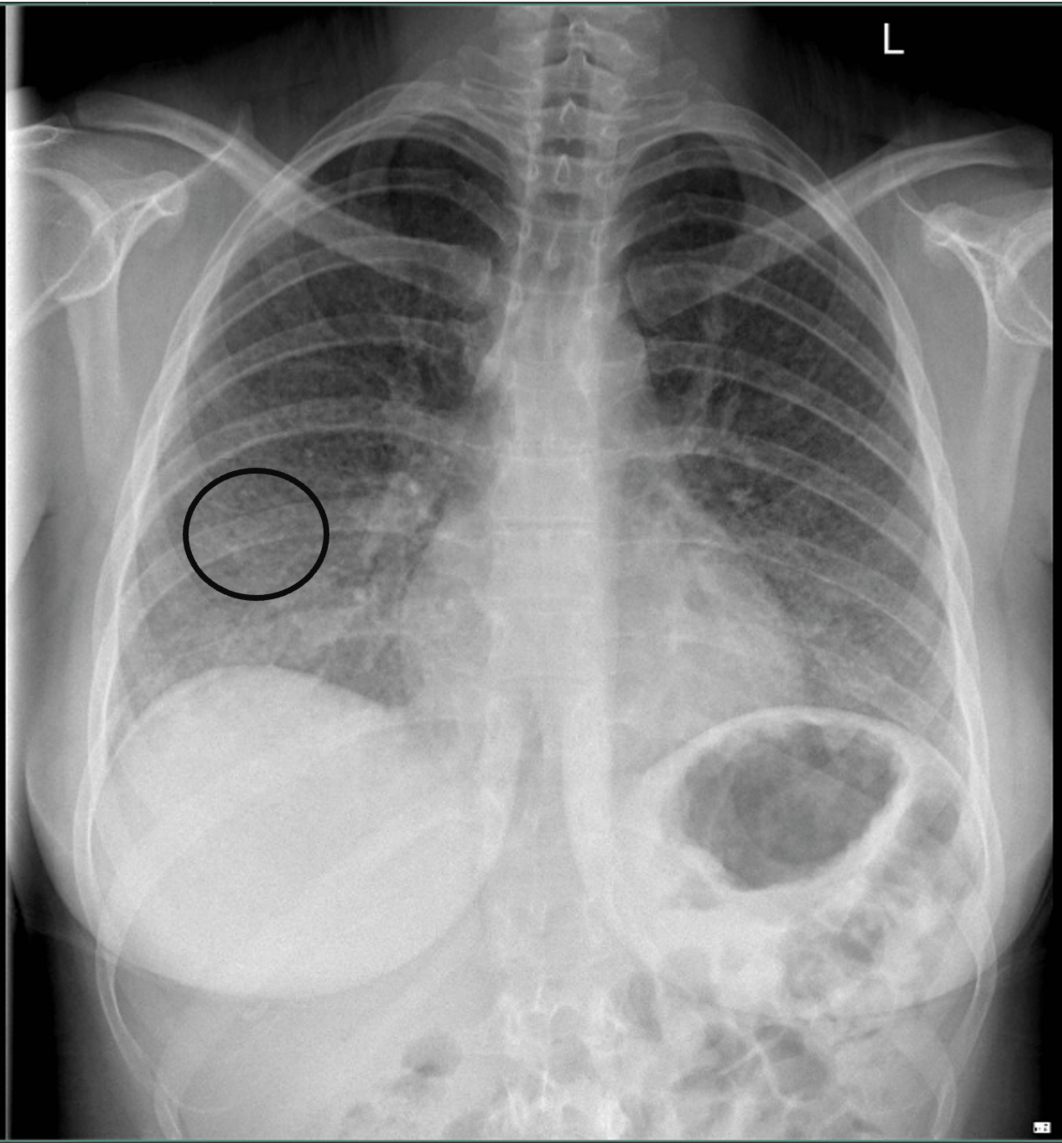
Admission chest X-ray. Nodular soft tissue pattern throughout both lung fields (best appreciated within circled area). No focal consolidation. Heart and mediastinal contours normal
